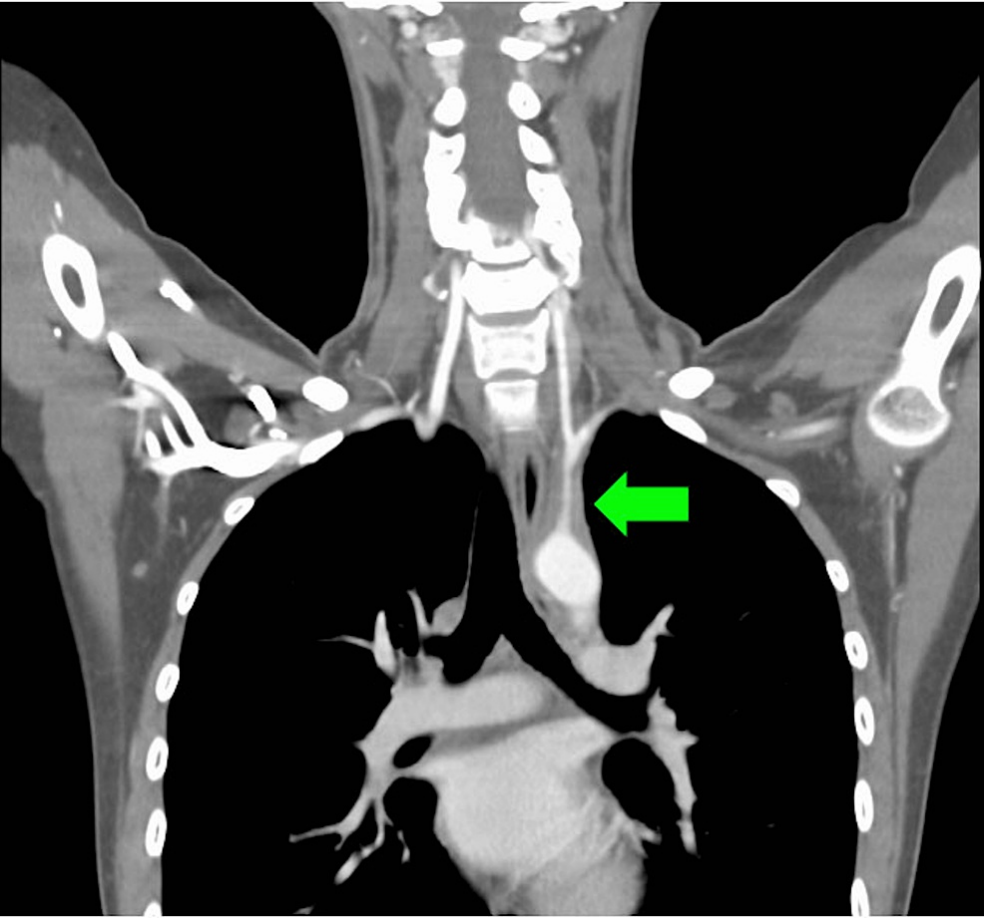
Contrast-enhanced computed tomography scan (sagittal view) exhibiting wall thickening in the left subclavian (green arrow) artery and its branches.
